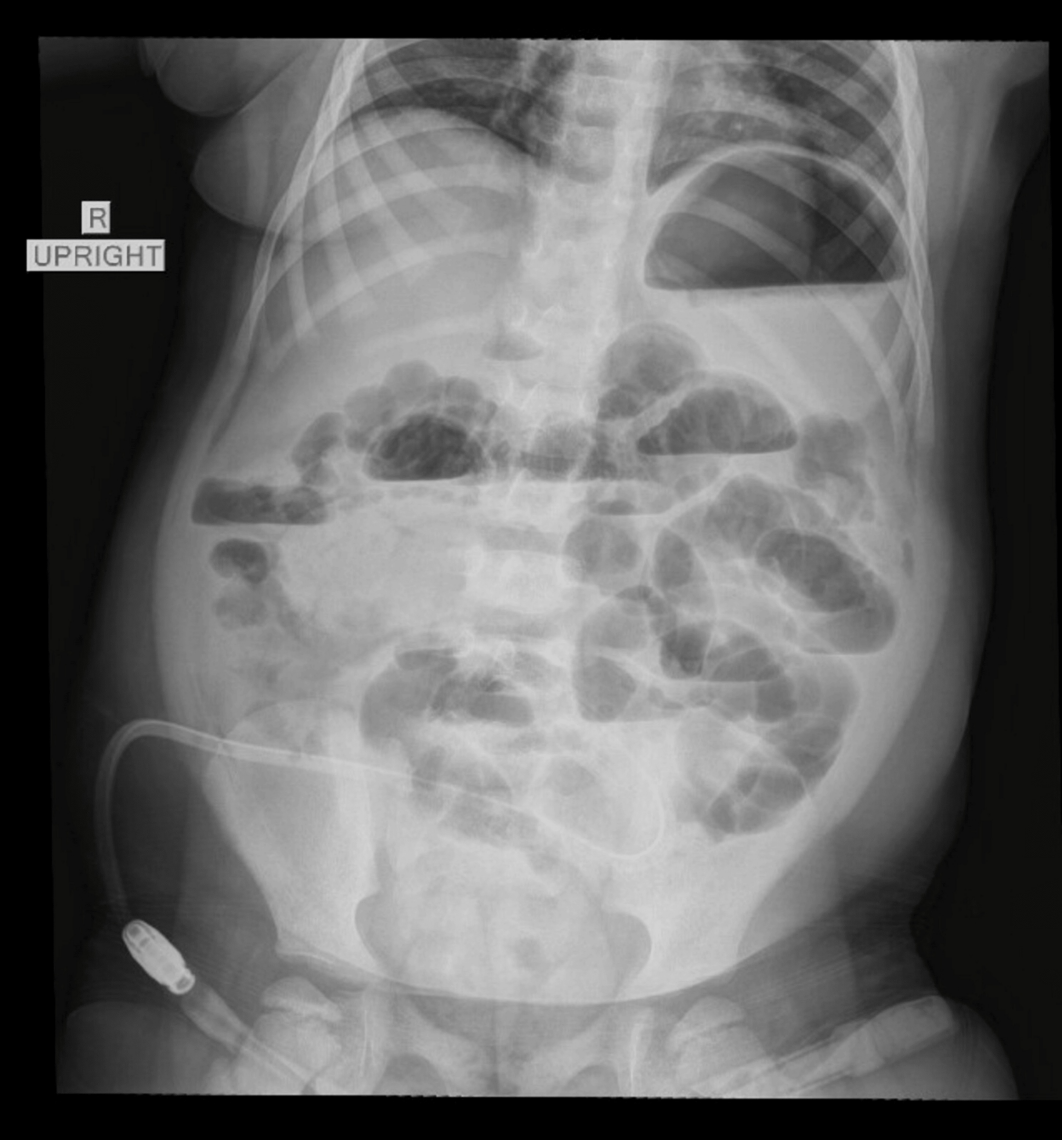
Abdominal X-ray showing multiple dilated loops of small bowel with air-fluid levels. The presence of a Tenkhoff catheter is visible.
